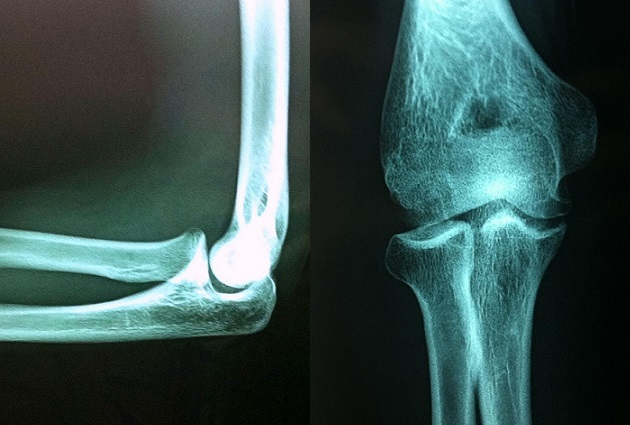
Foyer de condensation sous-chondral du capitellum: objectivé à la Radiographie du coude droit
